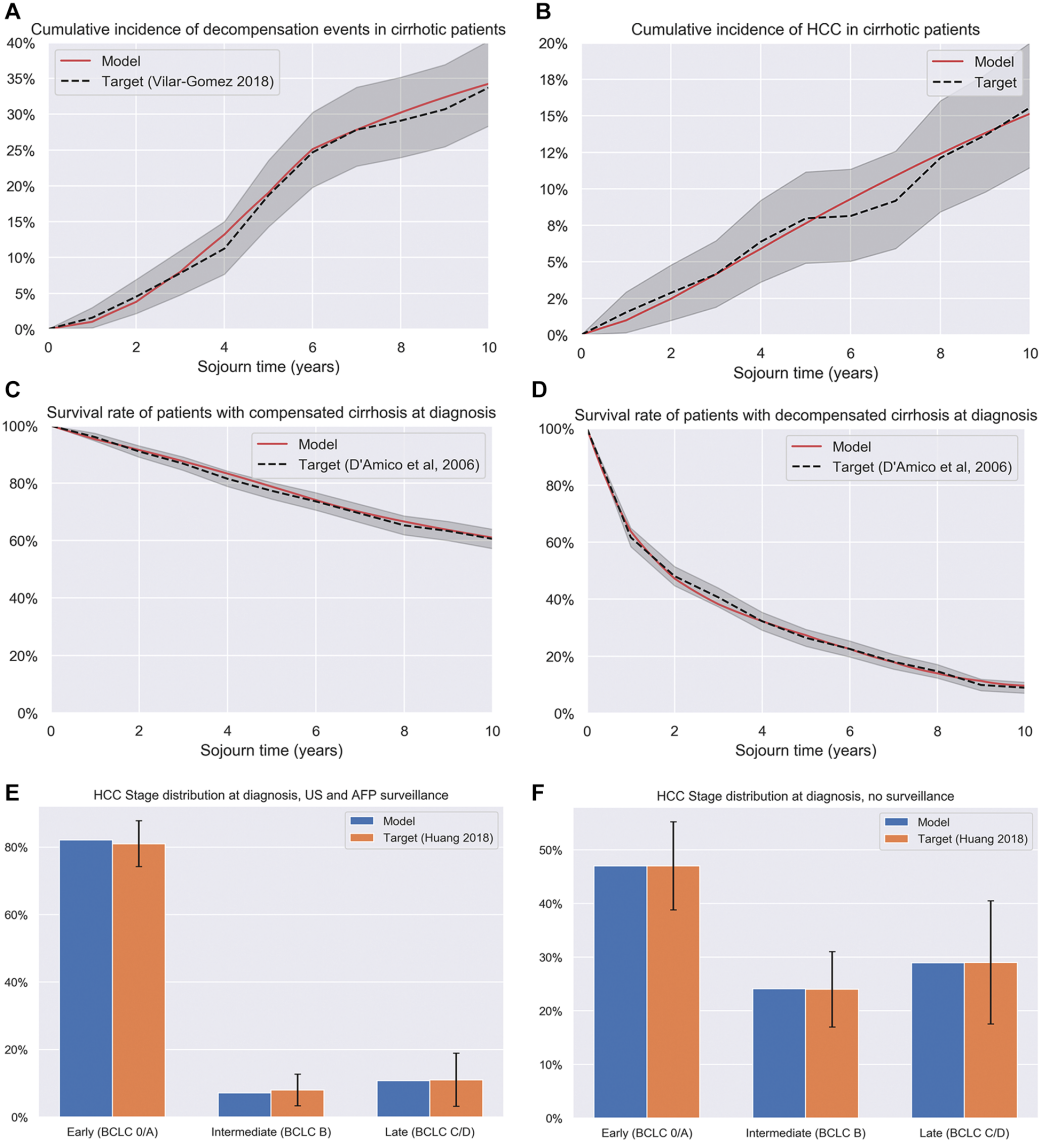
Selected calibration targets for the HCC model. AFP, alpha-fetoprotein; BCLC, Barcelona Clinic Liver Cancer; HCC, hhepatocellular carcinoma; US, ultrasound.
